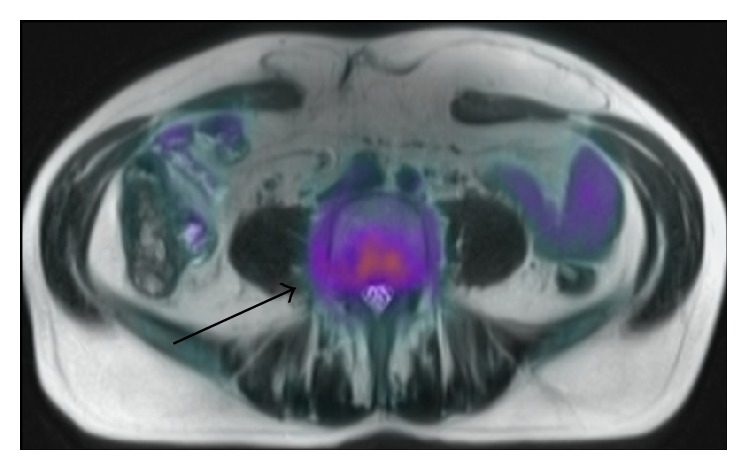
CT-PET axial segment showing high uptake in the paravertebral soft tissue regions at L4/L5.
